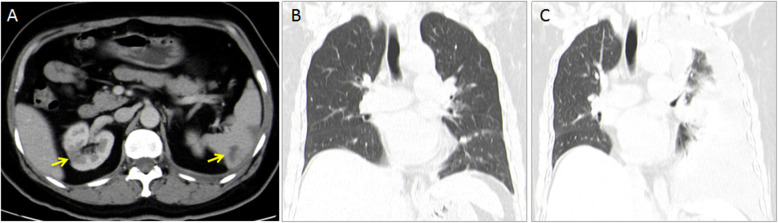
Abdominal CT scan revealed multiple infarction in spleen and kidney (a). The CT scan of chest showed increased pleural effusion (c) than that of a month ago (b)
